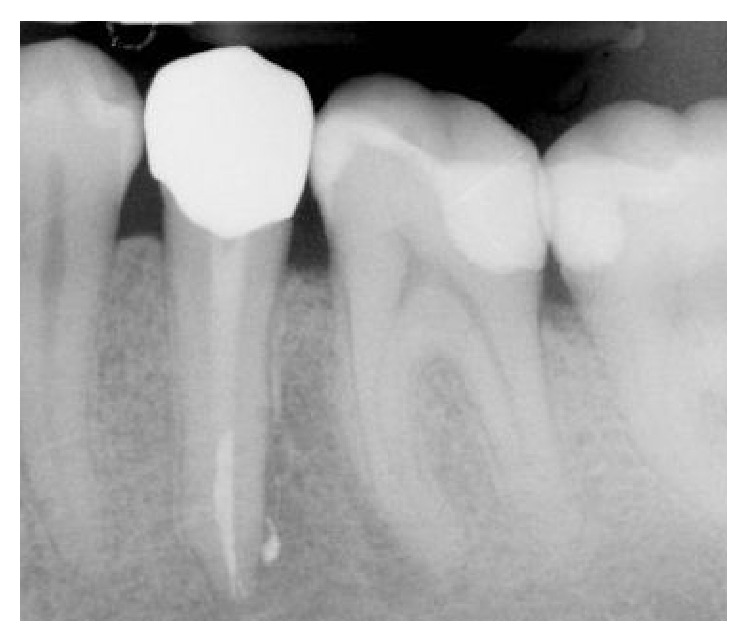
Radiographic control at one-year follow-up.
